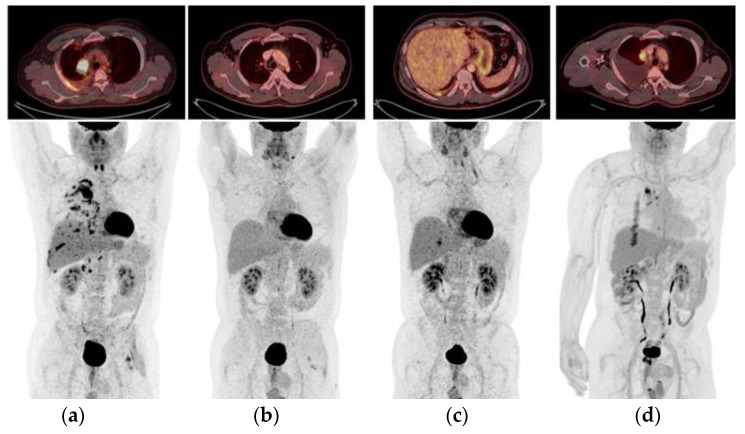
The pictures present four assessments performed during treatment of case 1. (a) Baseline positron-emission tomography (PET)/computed tomography (CT): A 5 cm 18F-Fluoro-deoxy-glucose (FDG)-avid tumor in the pulmonary upper right lobe and extensive metastatic spread including multiple small lesions in both lungs, multiple right-sided pleural lesions, mediastinal and right hilar lymph nodes, and several retroperitoneal lymph nodes. (b) After 6 months: PET/CT shows complete metabolic and considerable structural regression of all prior lesions. (c) At 9 months, control PET/CT shows, posteriorly, on the right side, an intense FDG uptake in relation to the pleura and pleural effusion, without any evident tumor seen on CT. The finding was suspicious for relapse. (d) Metabolic and structural progression of primary tumor in the right lung. Malignant mediastinal and right hilar lymph nodes. Increased metabolic activity posteriorly in the increasing right-sided pleural effusion—suspicion of malignant pleural effusion.
